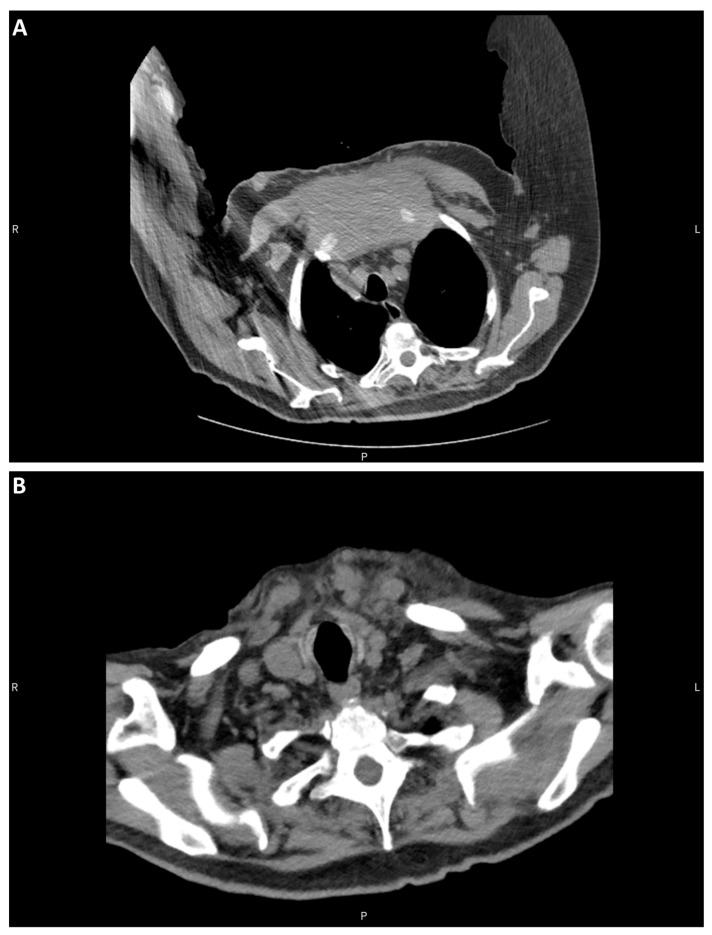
CT of the chest and neck. (A) Sternal mass. (B) Atrophic thyroid bed.
